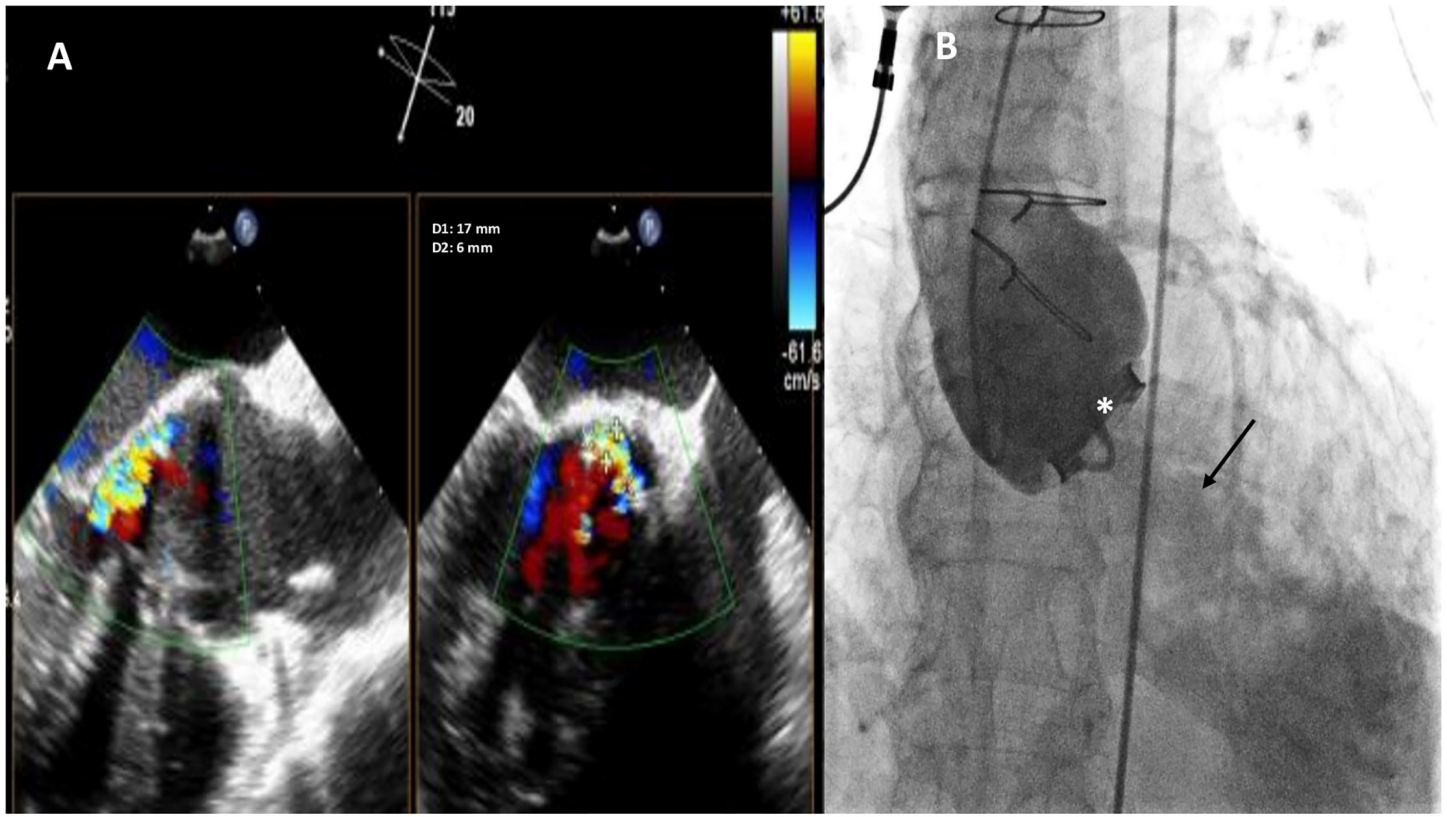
Baseline two-dimensional (2D) X-plane imaging Transoeophageal Echocardiogram (TEE) color Doppler (A) and ascending aorta angiography (B) showing the presence of a huge crescent-shaped 17 × 6 mm aortic PVL located at non coronary cusp (NCC) 9-12 o'clock, with moderate-to-severe regurgitation. Fluoro-angiographic image confirming the stable position of the mechanical tilting disc aortic prosthetic valve (white asterisk) with moderate regurgitation (black arrow).
